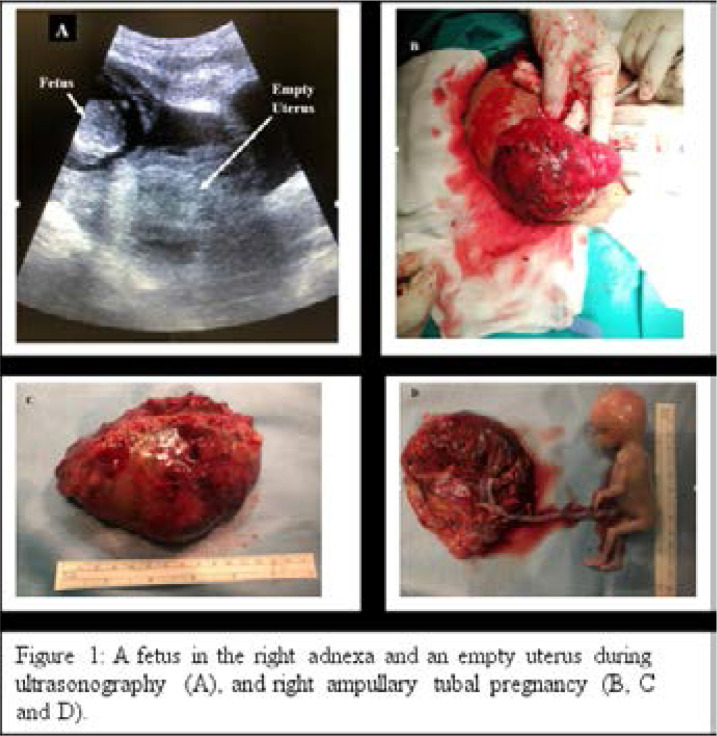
A fetus in the right adnexa and an empty uterus during ultrasonography (A), and right ampullary tubal pregnancy (B, C and D).
